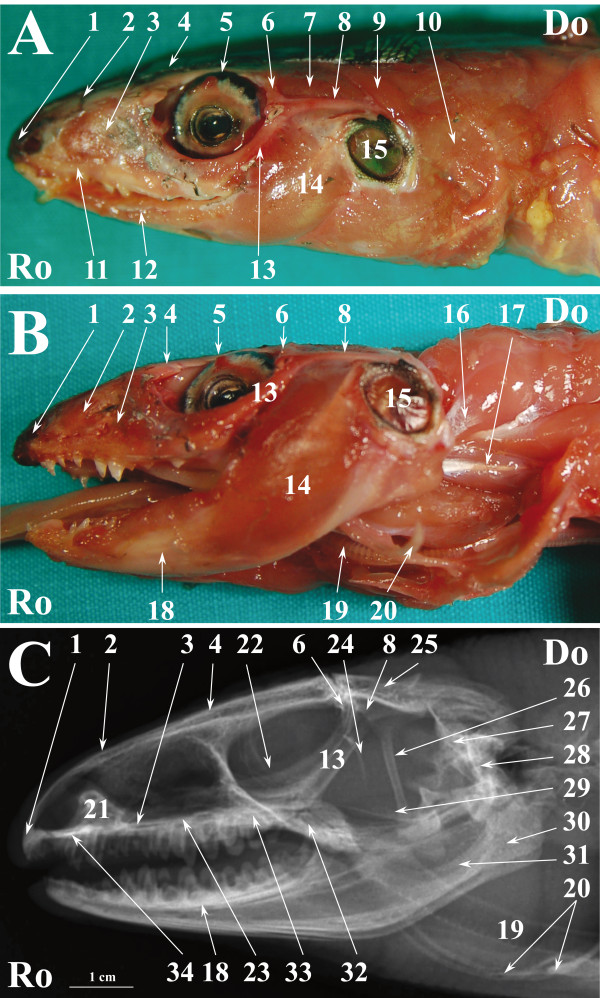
Normal radiographic anatomy of the head of the common tegu in LL projection.A. LL photographic image in a superficial plane of the stratigraphic dissection of the head of a tegu (only skin was removed). B. LL photographic image in a deep plane of the stratigraphic dissection of the head of a tegu C. LL radiographic projection of the head of a tegu. Ro is rostral, Do is dorsal. 1. Premaxillary bone; 2. Nasal bone; 3. Maxillary bone; 4. Frontal bone; 5. Eye; 6. Postorbital bone; 7. Musculus adductor mandibulae externus medialis; 8. Squamosal bone; 9. Musculus depressor mandibulae; 10. Musculus constrictor colli; 11. Supralabial glands; 12. Infralabial glands; 13. Jugal bone; 14. Musculus adductor mandibulae externus superficialis; 15. Ear; 16. Musculus obliquus capitis magnus; 17. Musculus spinalis; 18. Dentary bone; 19. Trachea; 20. Hyobranchial skeleton; 21. Septomaxilla; 22. Scleral ossicles; 23. Palatine bone; 24. Pila metoptica; 25. Parietal bone; 26. Epipterygoid bone; 27. Occipital bone; 28. Quadrate bone; 29. Rostrum parasphenoid; 30. Articular bone; 31. Angular + Surangular bone; 32. Pterygoid bone; 33. Coronoid bone; 34. Vomer bone.
